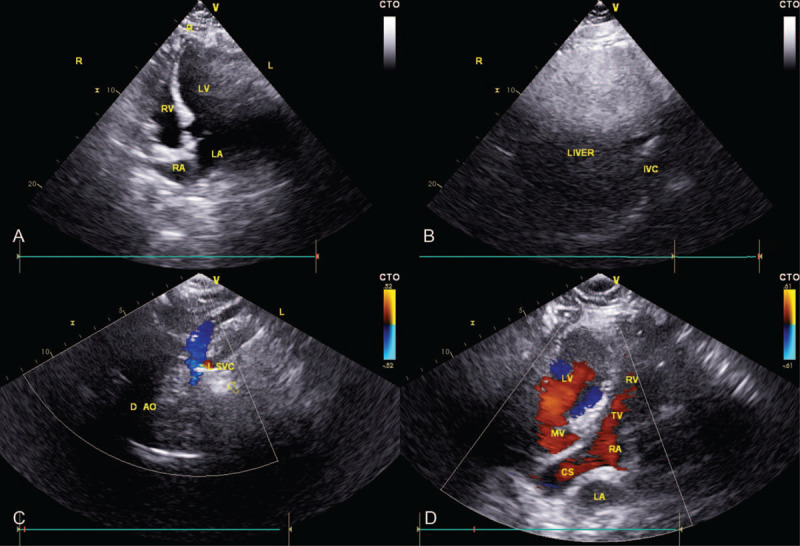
Cardiac color Doppler echocardiography: dextrocardia, live at the same time with the left superior vena cava lead into the right atrium by expanding coronary sinus.
